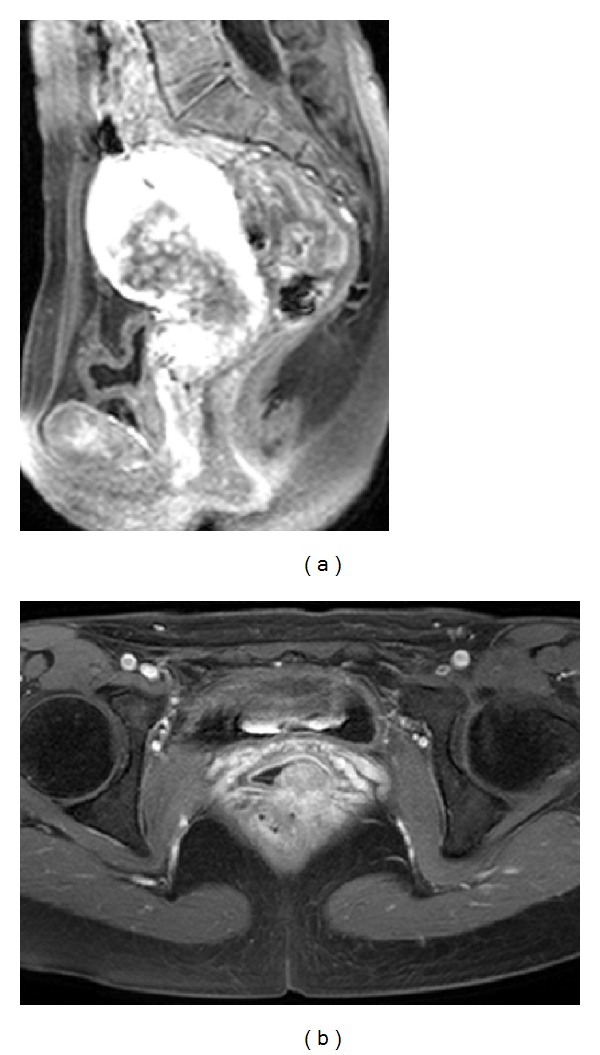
Pelvic MRI. (a) Sagittal fat-suppressed T1-weighted MR image after gadolinium administration showed region heterogeneously poor enhanced in cervix, vagina, and uterine corpus myometrium which were irregularly enhanced in late phase. (b) Axial fat-suppressed T1-weighted MR image after gadolinium administration showed cervical mass homogeneously enhanced in the posterior wall from the uterine cervix to the vagina.
